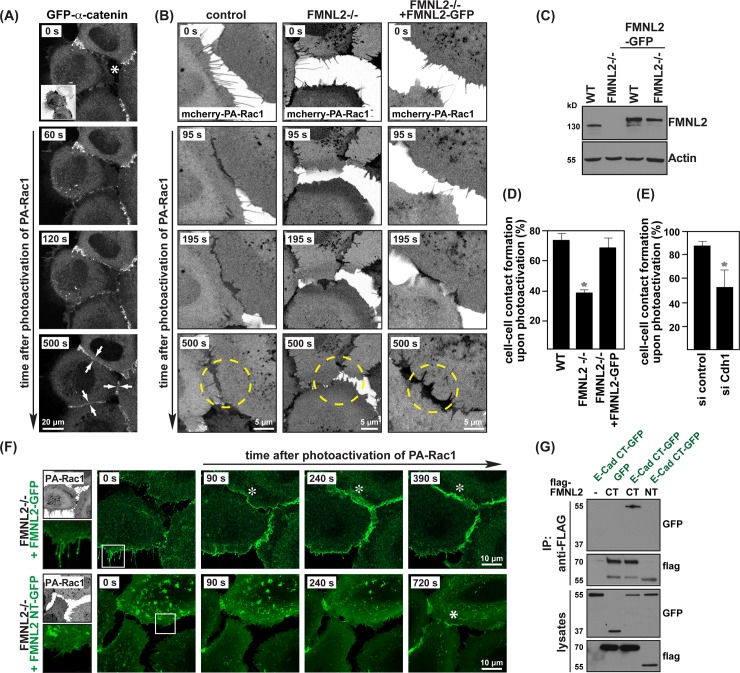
Impairment in cell-cell contact formation in FMNL2-/- cells can be rescued by reintroduction of FMNL2-GFP. (A) Live wildtype MCF10A cells expressing mCherry-PA-Rac1 and GFP-α-catenin were imaged over time to visualize adhesion junction formation upon Rac1 activation. Small inset shows mCherry-PA-Rac1 expression. (B) Comparison of cell-cell contact formation dynamics upon PA-Rac1 activation in indicated cell lines. Dashed circles highlight regions of interest. (C) Western blot comparing endogenous FMNL2 and exogenous FMNL2-GFP expression. Antibodies were applied as indicated. (D) Quantification of cell-cell contact establishment after Rac1 activation as in (B) (*n* = 60 (WT), *n* = 65 (FMNL2-/-), *n* = 64 (FMNL2-/- + FMNL2-GFP), pooled from three independent experiments, error bars SD, *p ≤0.05,). (E) Quantification of cell-cell contact formation in cells treated either with control or Cdh1 siRNA (*n* = 43 (si control), *n* = 44 (si Cdh1) pooled from three independent experiments, error bars SD, *p≤0.5 calculated by *t*-test). Western blot demonstrating siRNA efficiency in MCF10A cells in S1E Fig. (F) Live FMNL2-/- cells co-expressing FMNL2-GFP or FMNL2-NT-GFP with PA-Rac1 (upper small image) were imaged. Stills show protrusion dynamics and contact formation over time. Zoom image depict magnification of filopodia. Asterisks highlight areas of contact sites. (G) Co-immunoprecipitation of HEK cells expressing GFP or E-Cadherin CT-GFP with flag-tagged FMNL2 derivatives. Flag-tagged proteins were precipitated and bound proteins were detected using GFP-HRP antibody. Please note, E-Cadherin CT-GFP is pulled down only with flag-FMNL2 CT. In immunoprecipitates of cells expressing high levels of flag-FMNL2 CT, a band at 60 kD is observed likely deriving from a truncation product.
